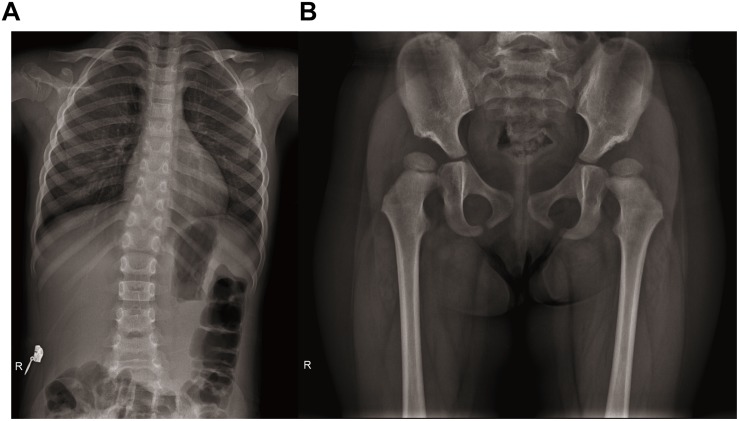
Radiographs of spine and hip joints. (A) The spine radiograph showing the patient has scoliosis. (B) Bilateral femoral head moved upward. Bilateral acetabular articular surface was flat and shallow. Bilateral Shen Tong’s line was discontinuous. Poor alignment of left hip joint.
